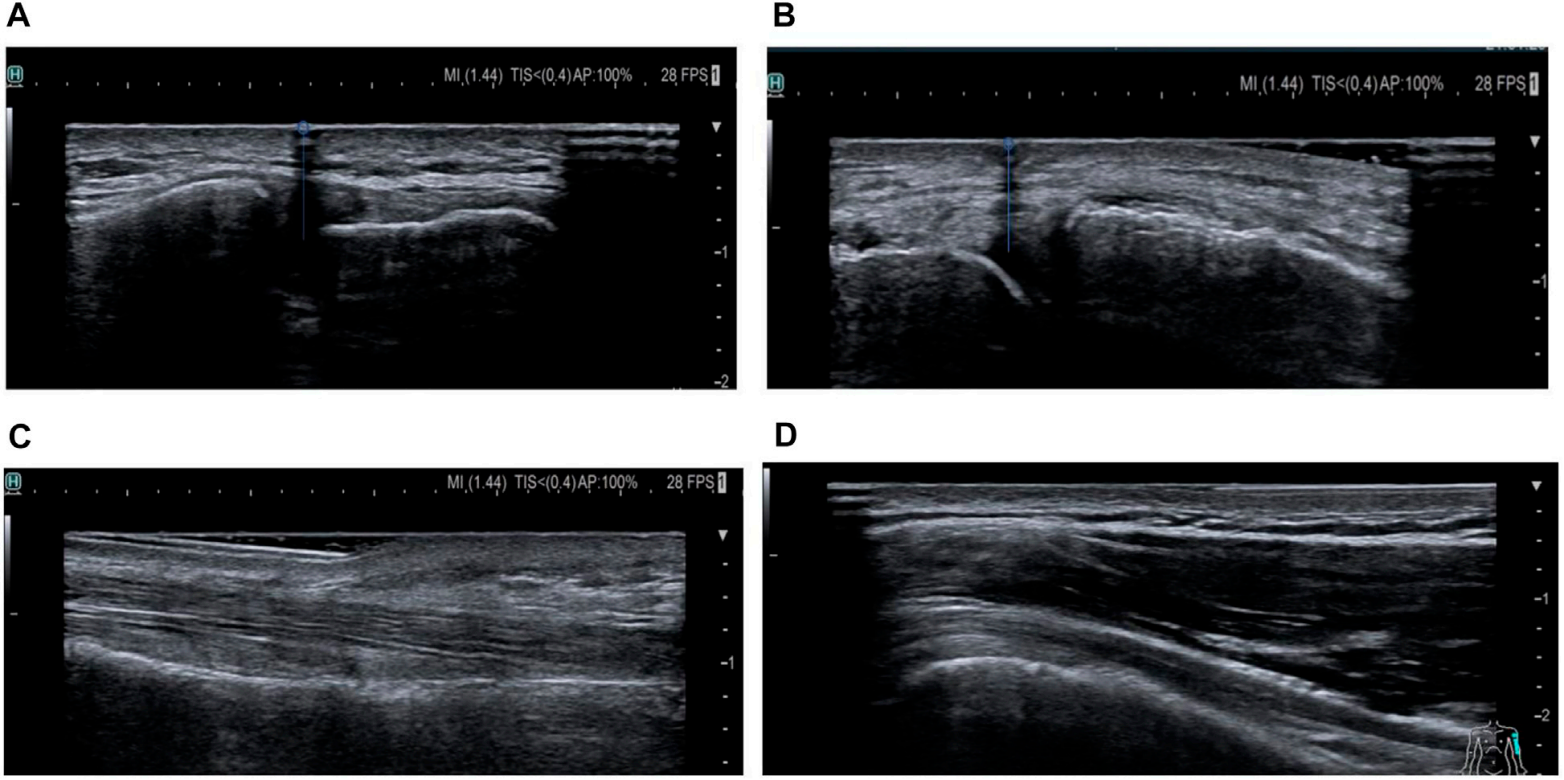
Needle placement based on target structures relative to ultrasound images. (A) Correct localization map of the ACJ. (B) Correct localization map of the TFJ. (C) Map of mislocalization of the long head of the biceps tendon. (D) Map of the mislocalization of the posterior tibialis muscle.
